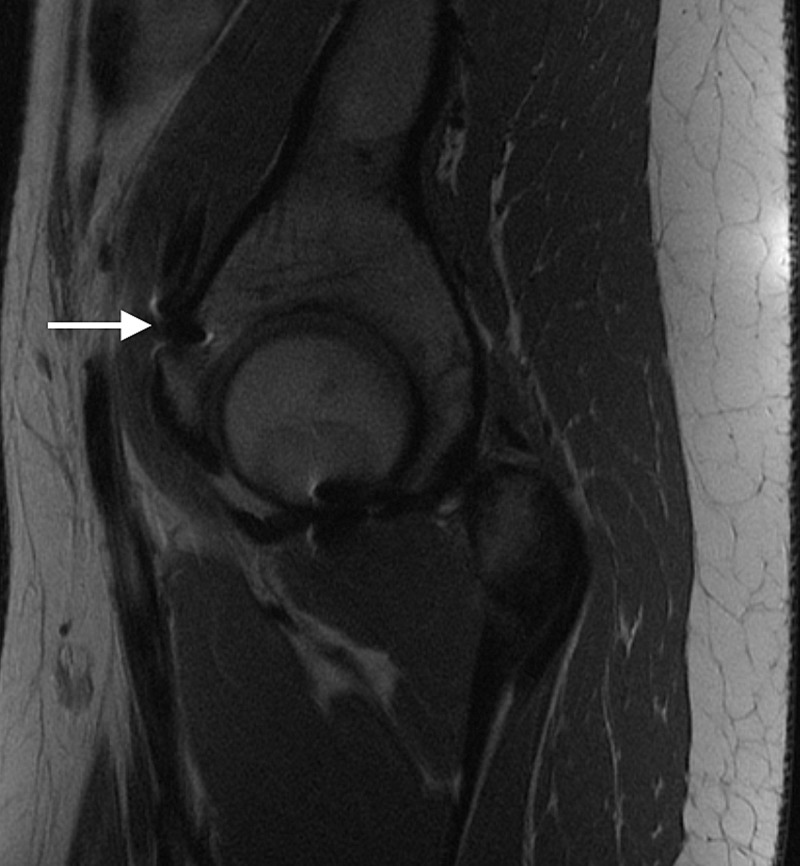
Sagittal MRI demonstrating medial cortical perforation with prominent suture anchor (white arrow) 65 × 70 mm.
